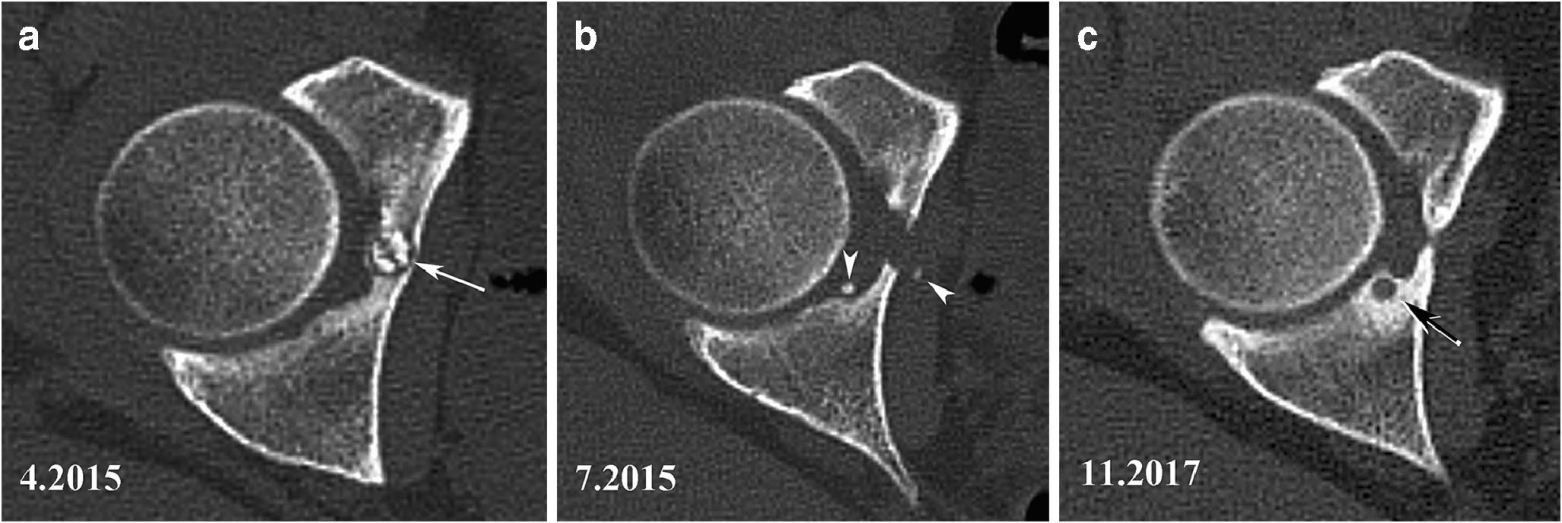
Recurrence near the original site of a resected OO. An OO involving the deeper aspect of the acetabulum (arrow in CT image in a) was arthroscopically resected. A CT examination performed after surgery (b) showed complete lesion resection and the presence of small bone fragments (arrowheads) near the resected site. The pain recurred after several months and repeated CT examination performed 30 months after surgery demonstrated appearance of a small lucent area surrounded by hyperostosis near the resection site (arrow in c). Recurring OO nidus was confirmed on DCE-MRI (not shown) and successfully electrocoagulated
